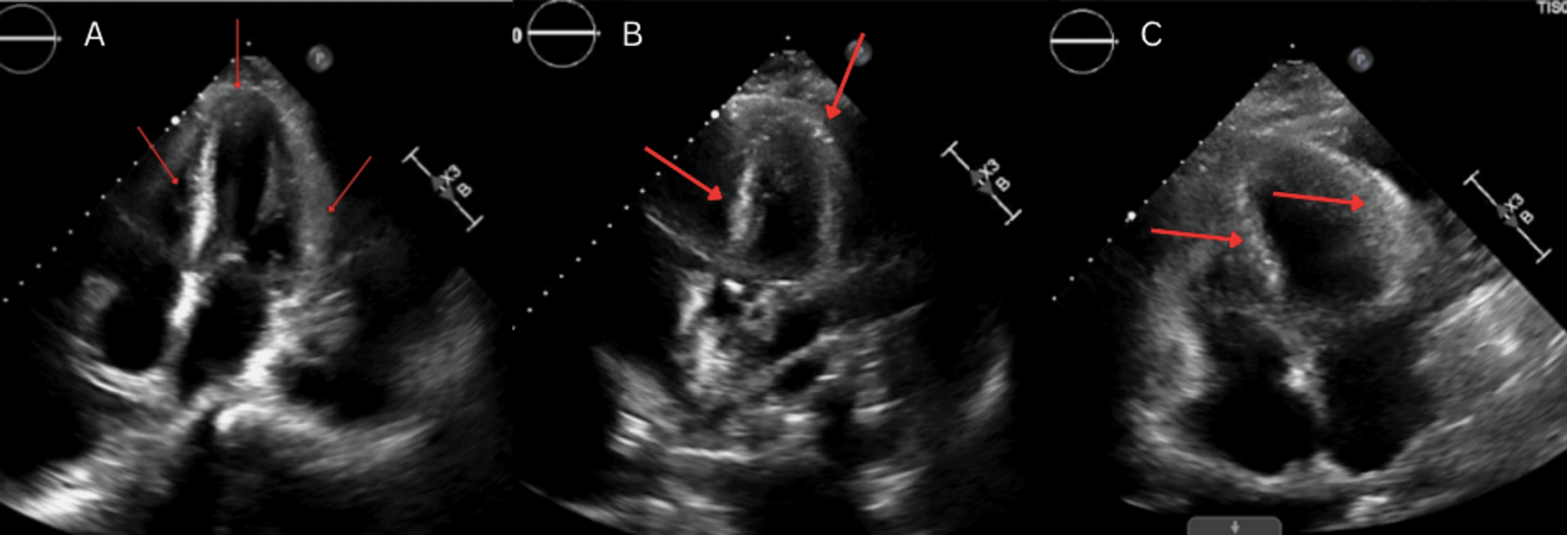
Transthoracic echocardiogram demonstrating findings consistent with cardiac amyloidosis Figure shows apical four-chamber views demonstrating concentric left ventricular hypertrophy and a characteristic “speckled” or granular myocardial appearance (red arrows). Strain imaging revealed an apical sparing pattern with preserved left ventricular ejection fraction (50-55%), classic echocardiographic features concerning for cardiac amyloidosis.
